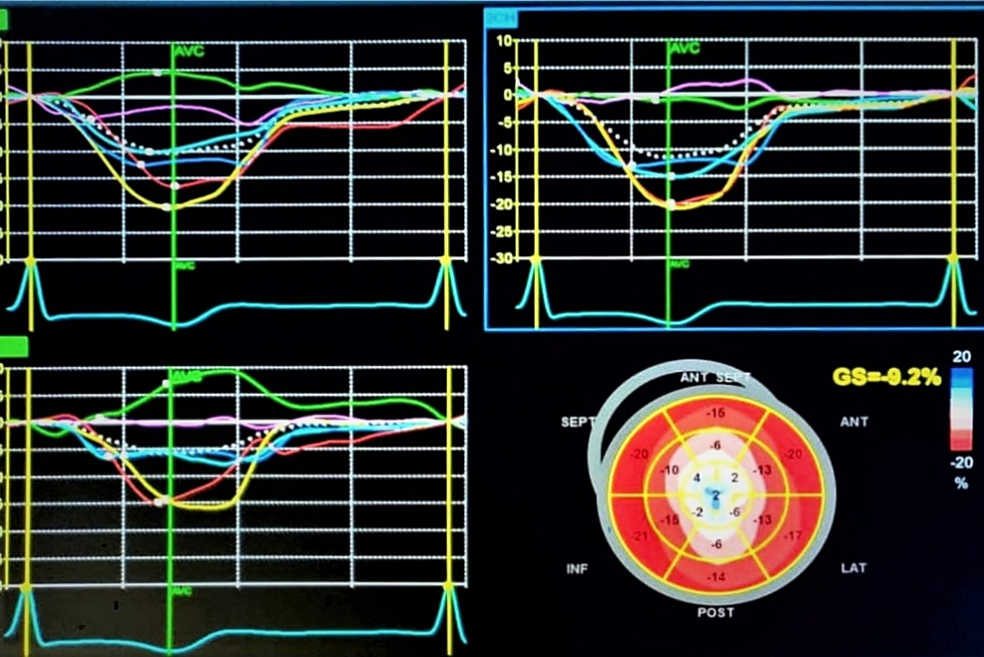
Left atrial strain of six segments as regions of interest depicted by different colours and overall PALS shown by white dots (A) Apical 4 chamber view; (B) Apical 2 chamber; (C) overall LA strain of all views where PALS is calculated at the end of T wave; (D) Bulls' eye representing various LA strain PALS: peak atrial longitudinal strain; LA: left atrium
